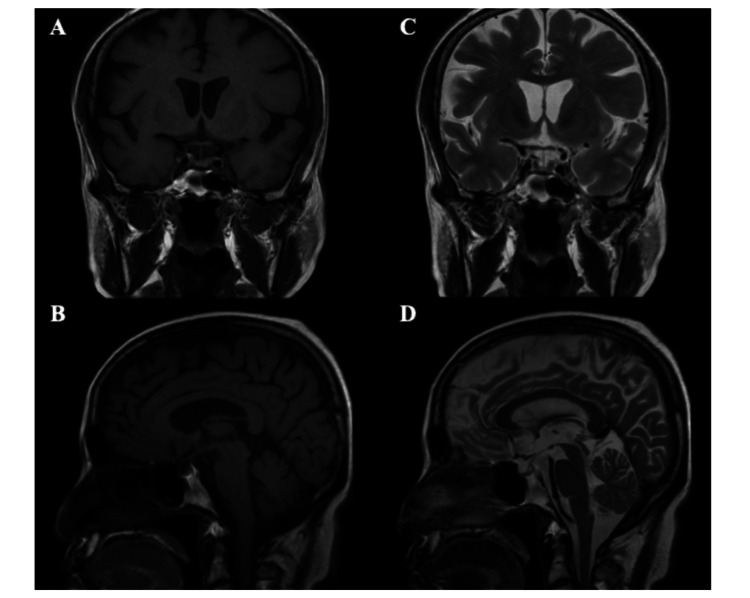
The pituitary MRI images The patient’s pituitary gland T1WI coronal (A) and axial (B) images and T2WI coronal (C) and axial (D) images are shown. No organic lesions were observed in the pituitary region. T1WI, T1-weighted images; T2WI, T2-weighted images
